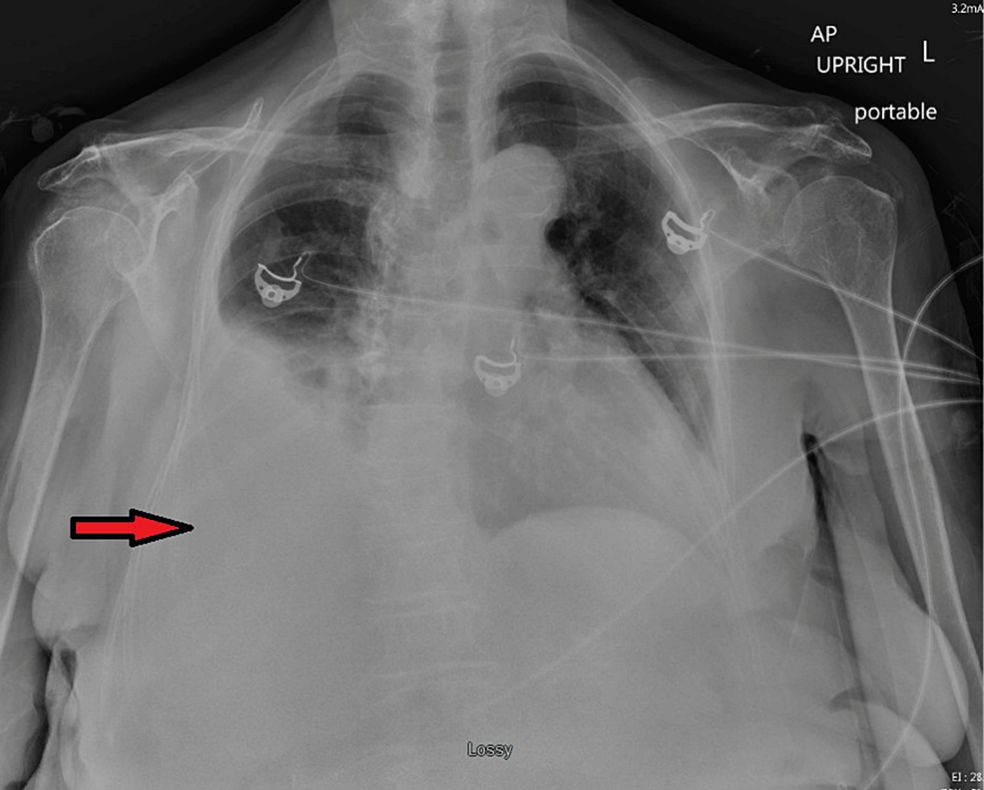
Chest x-ray showing right pleural effusion.
